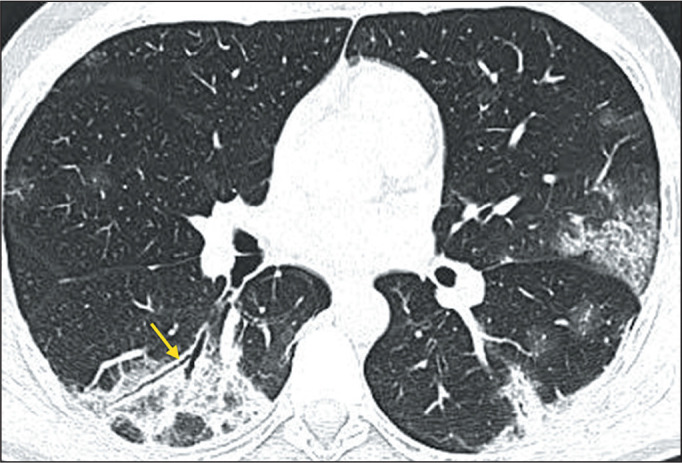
CT scan of a 39-year-old female patient, showing ground-glass opacities
